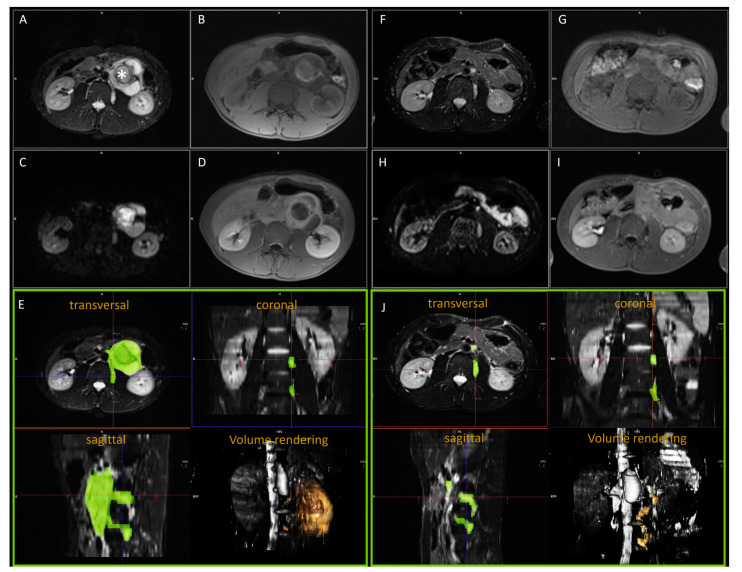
Example of imaging analysis in a 15-year-old male patient with ganglioneuroblastoma. (A–E) Before and (F–J) after resection. (A,F) T2 weighted images with fat saturation. (B,G) Non-enhanced T1 weighted images and (D,I) after application of contrast medium. (C,H) DWI with high b-value (ADC map not shown). (E,J) The multiplanar reconstruction respective 3D volume analysis; the tumor volume is colored green, and in the volume rendering method it is colored light yellow. Before resection, the tumor located anterior to the spine reached the left renal hilum and had extensions into the ipsilateral neuroforamina. MRI characterized the tumor as inhomogeneous with hemorrhage (star), inhomogeneous to even absent contrast enhancement. There was a moderate diffusion restriction in the area of the non-regressively altered tumor parts. Tumor volume was 234 mL. After resection, two extensions could be detected unchanged in the neuroforamina and a small RD adjacent to the aorta. The total volume was 11 mL. According to the surgical report, the extensions into the neuroforamina were not resected (IME) because this patient did not have a high-risk situation. There was no tumor progression.
